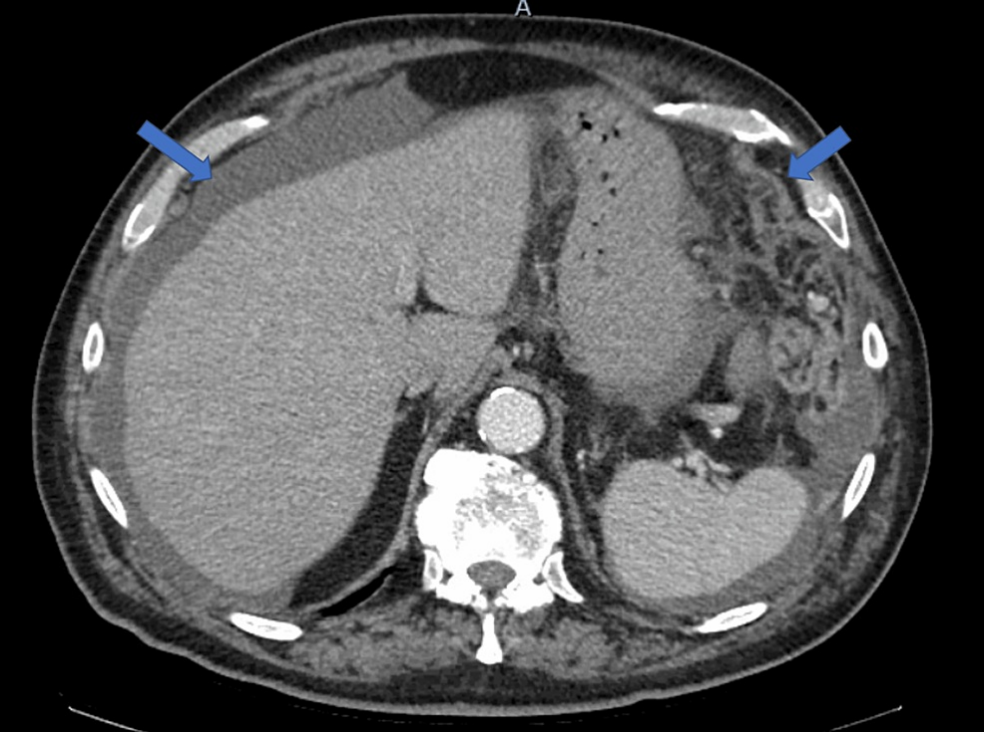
Abdominal ascites and nodularity of the omentum suggestive of peritoneal carcinomatosis seen on chest CT on December 28, 2020
